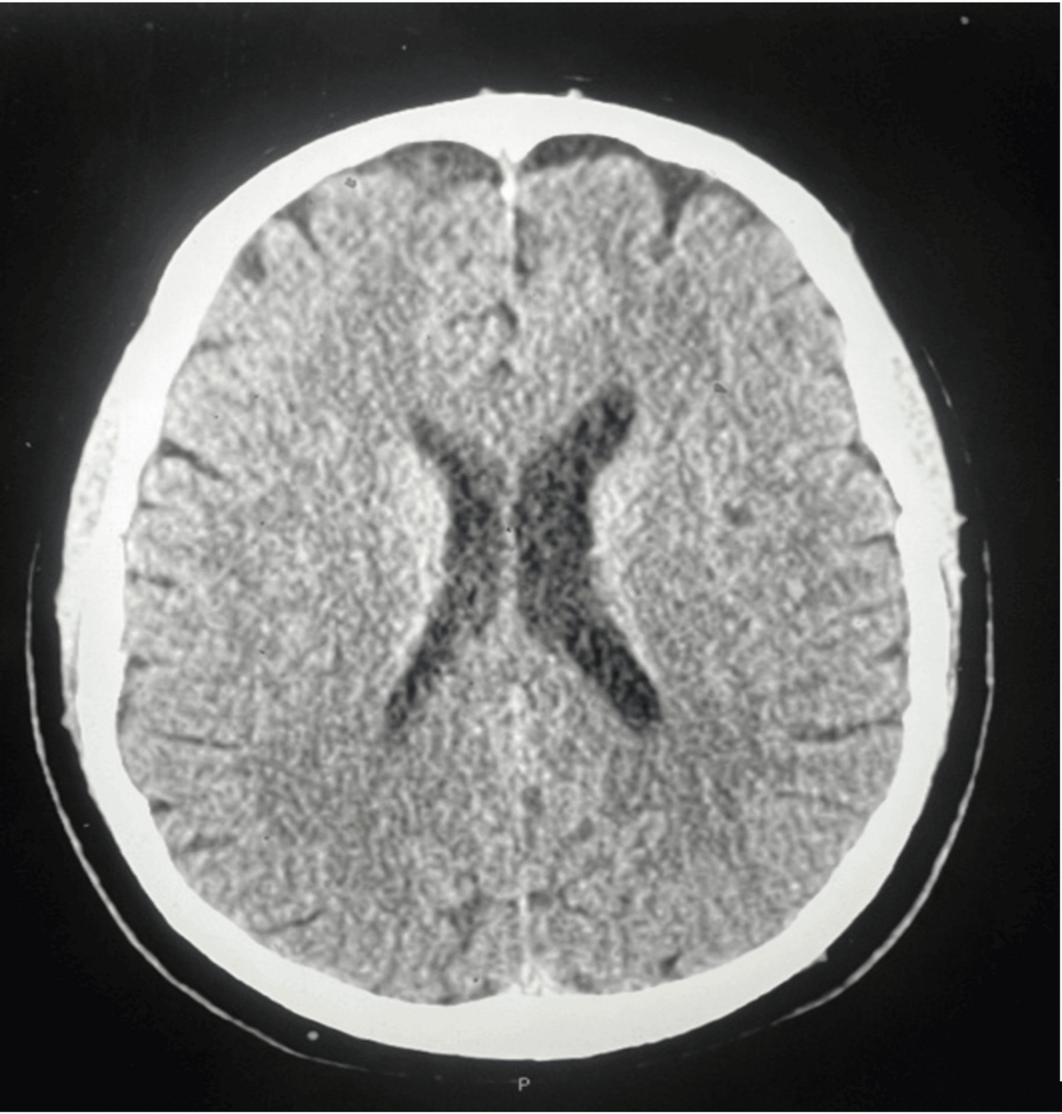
CT scan of the patient's brain No acute intracranial abnormalities
